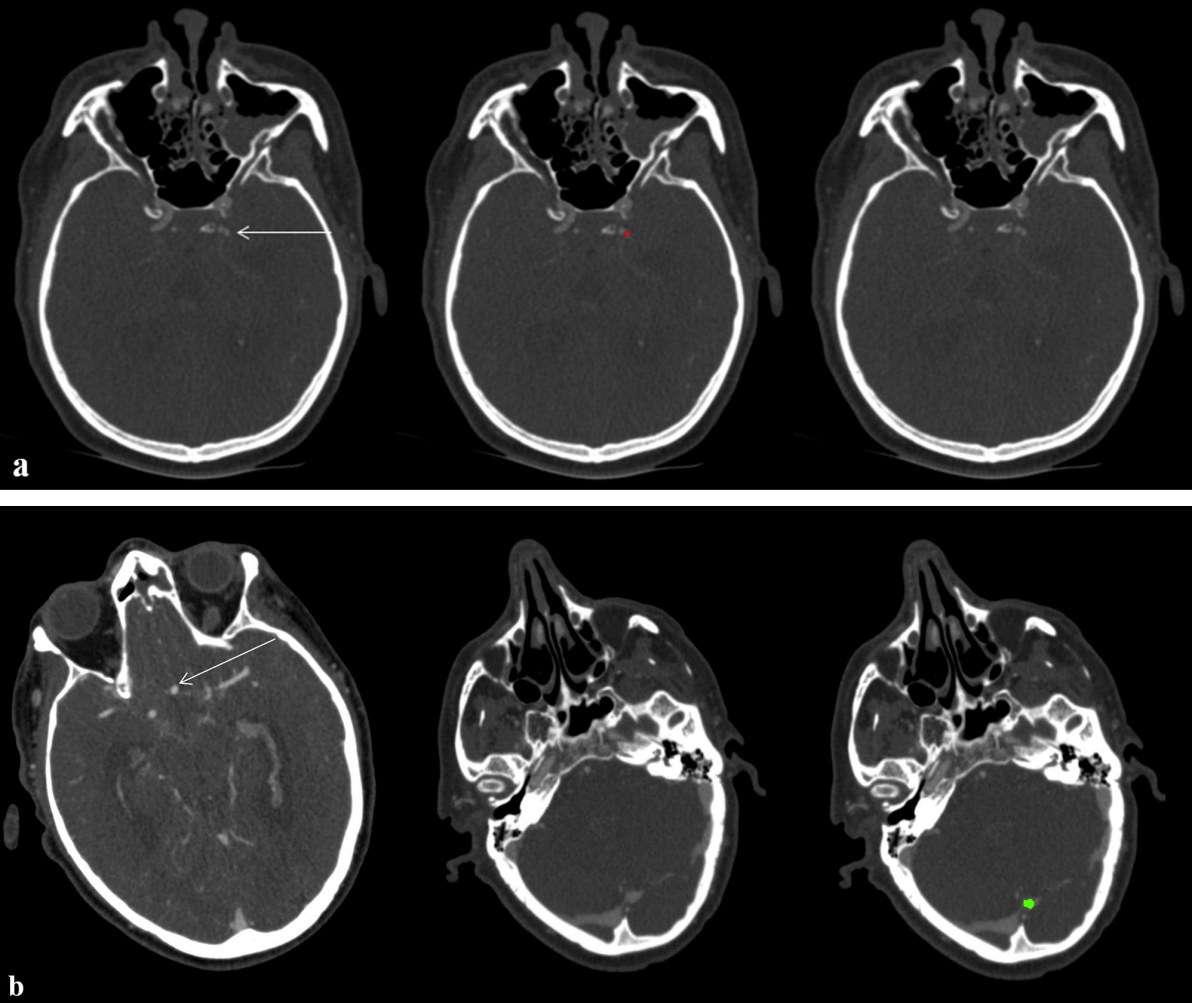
(a) Axial CTA source images of a 64-year-old male with a small aneurysm (volume based on manual segmentation: 21.8 mm^3^) of the left internal carotid artery (arrow, red: manual segmentations) being missed by the DLM-Ens. (b) Axial CTA source images of a 65-year-old male with an anterior communicating artery aneurysm (arrow), which was accurately segmented and detected by the DLM. However, a false positive finding of the DLM-Ens (in green) at the superior sagittal sinus/transverse sinus was detected.
